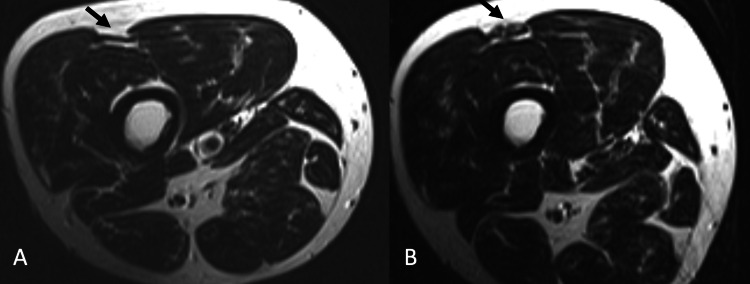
Dynamic MRI evaluation of the fascia defect showed with arrow both in relaxation (A) and contraction (B)
